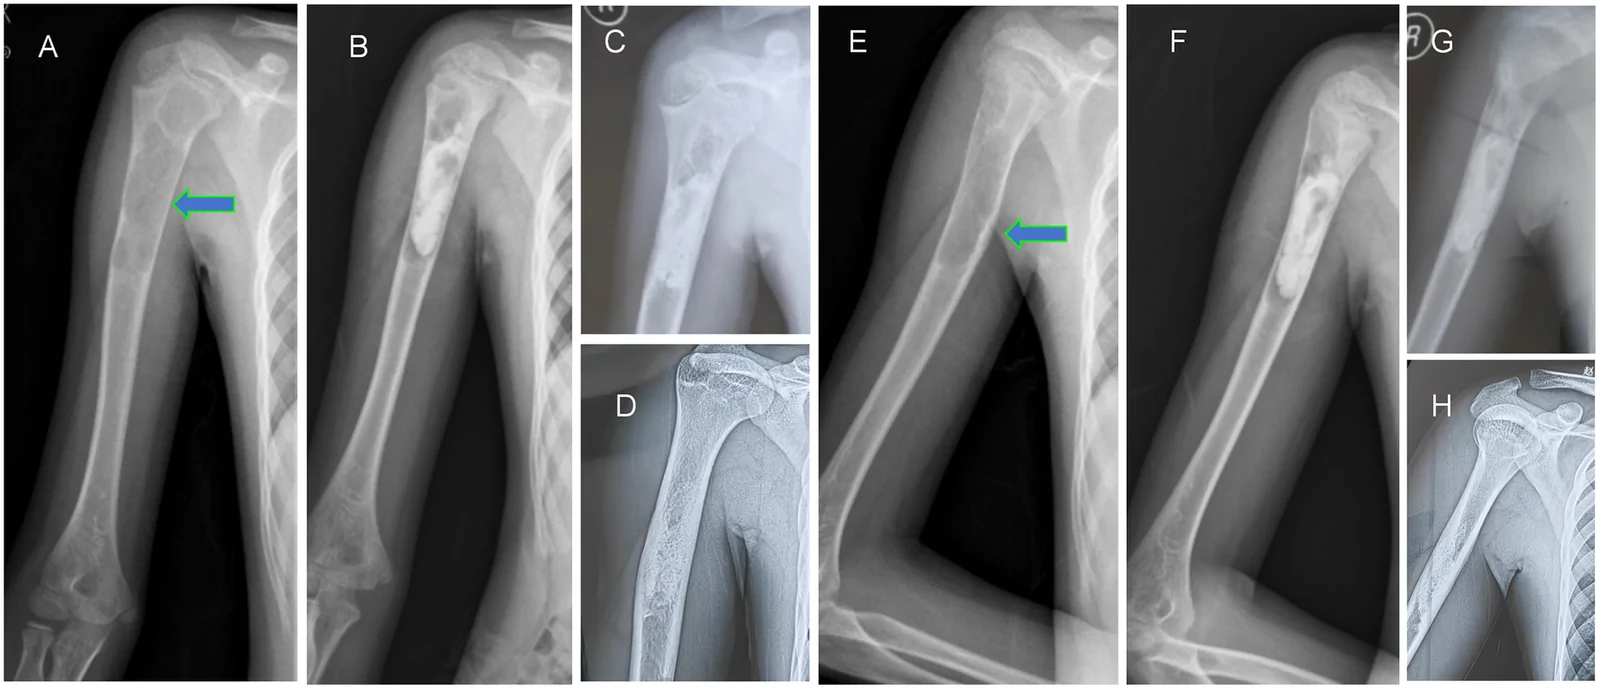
Representative radiographic changes in patient 8 after a single percutaneous CPC injection. (A) Preoperative anteroposterior (AP) radiograph showing the lesion, indicated by the arrow. (B–D) Postoperative AP radiographs obtained at 3 months, 1 year, and 7 years after injection, respectively. (E) Preoperative lateral radiograph showing the lesion, indicated by the arrow. (F–H) Postoperative lateral radiographs obtained at 3 months, 1 year, and 7 years after injection, respectively. Progressive cavity consolidation and cortical remodeling were observed during follow-up.
